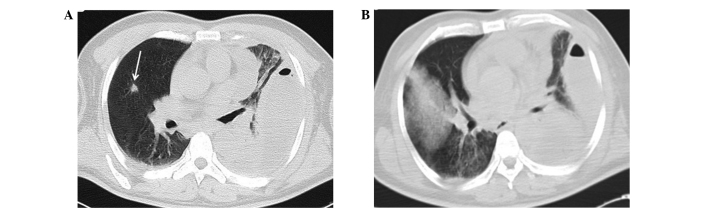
CT scan of a 54-year-old male. (A) Small nodule (arrow) in the right upper lobe with pleural effusion in the left thoracic cavity, demonstrated to be adenocarcinoma by biopsy and surgery. (B) Immediately after biopsy, a moderate hemorrhage with patchy haziness around the lesion was noted.
